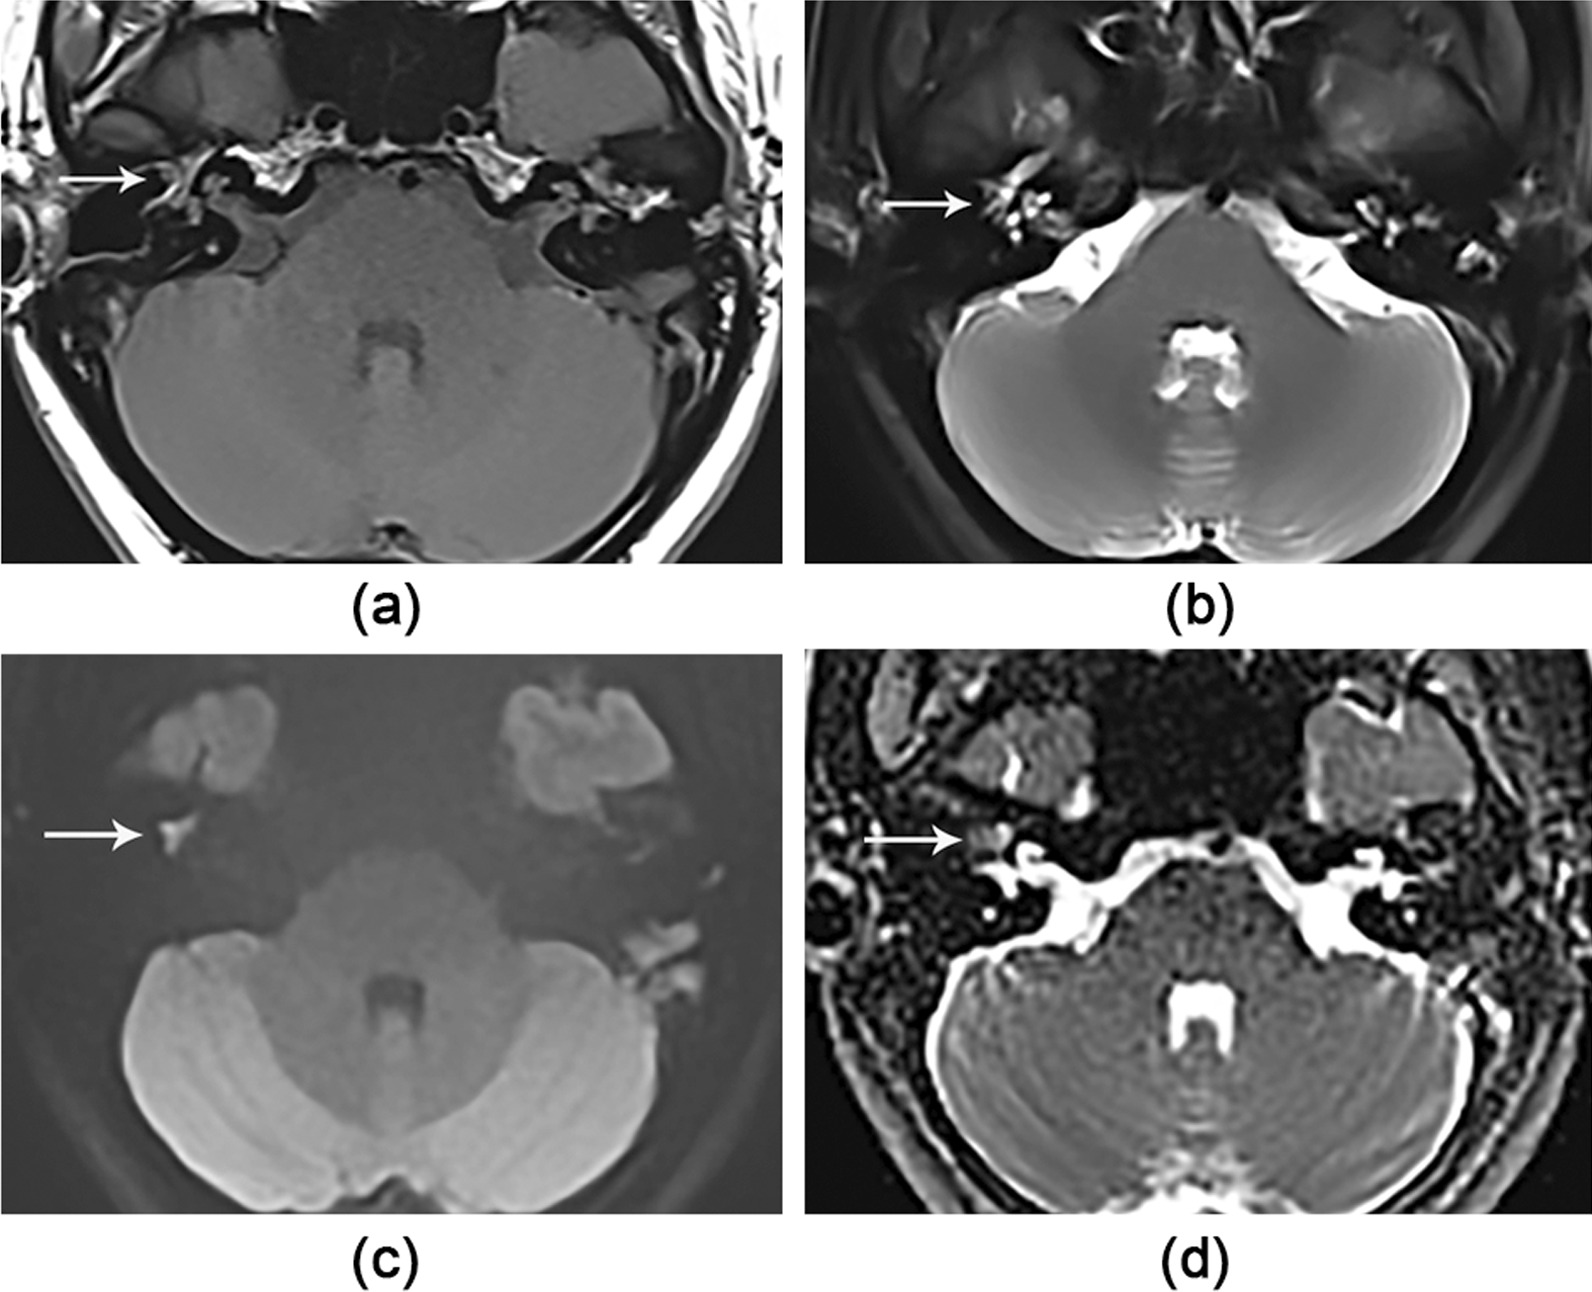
A 22-year-old female patient presented with true-positive CS of 2.4 mm in the right side. Axial images show the lesion (white arrow) as high signal on (b) the T2WI-FS, (c) TGSE BLADE DWI b1000 image and (d) ADC map, and an iso-intensity on (a) the T1WI compared to brain tissue
